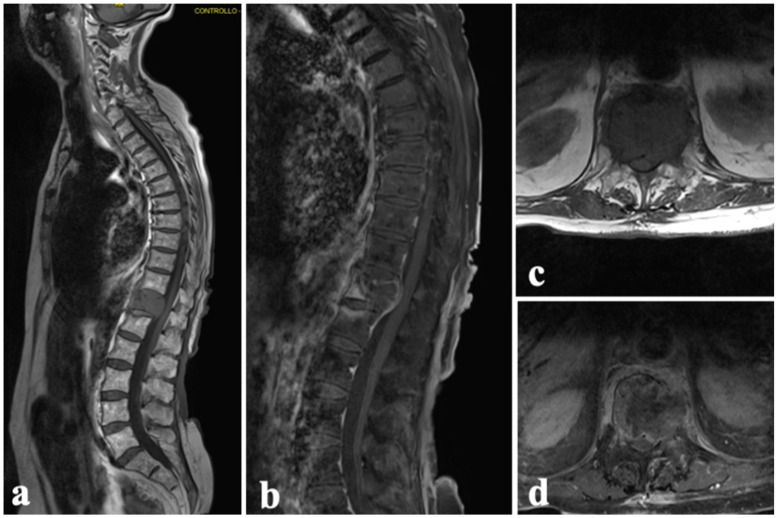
Case 2. A 76-year-old woman with T12 metastasis from squamous cell carcinoma: (a,b) preprocedural MRI in sagittal and (c,d) axial plane showing the complete involvement of anterior vertebral body and partial cord compression.
